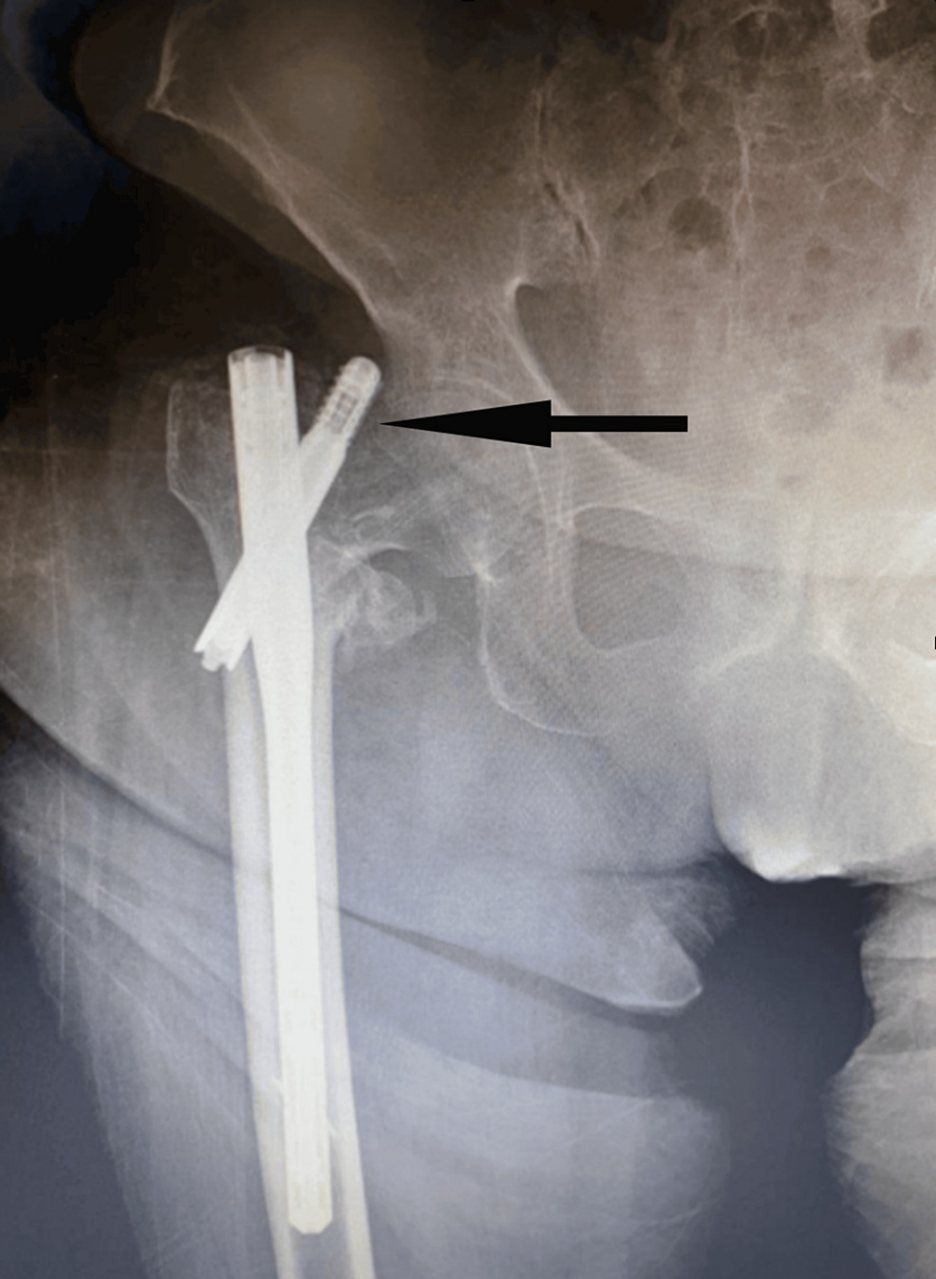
Postoperative 15th day X-ray radiograph of the patient who developed failure because of the failure of the lag screw Talon-lock mechanism (black-arrowed area)
